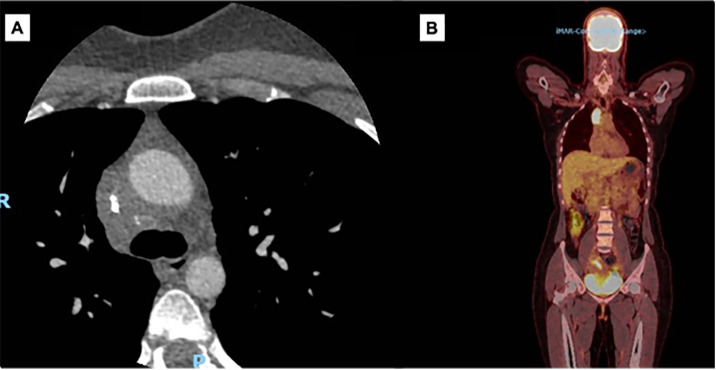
A. A 31-year-old woman with no comorbidities presented with facial plethora and oedema of the cervical region and upper limbs. A CT angiography scan revealed a lesion measuring 5.4 × 3.0 × 4.4 cm, with approximately 75% reduction in the caliber of the superior vena cava. She was diagnosed with pulmonary histoplasmosis after positive serology results obtained by two different methods. The mediastinal biopsy revealed only fibrosis. B. PET/CT scan showed a mediastinal mass with high glycolytic activity.
